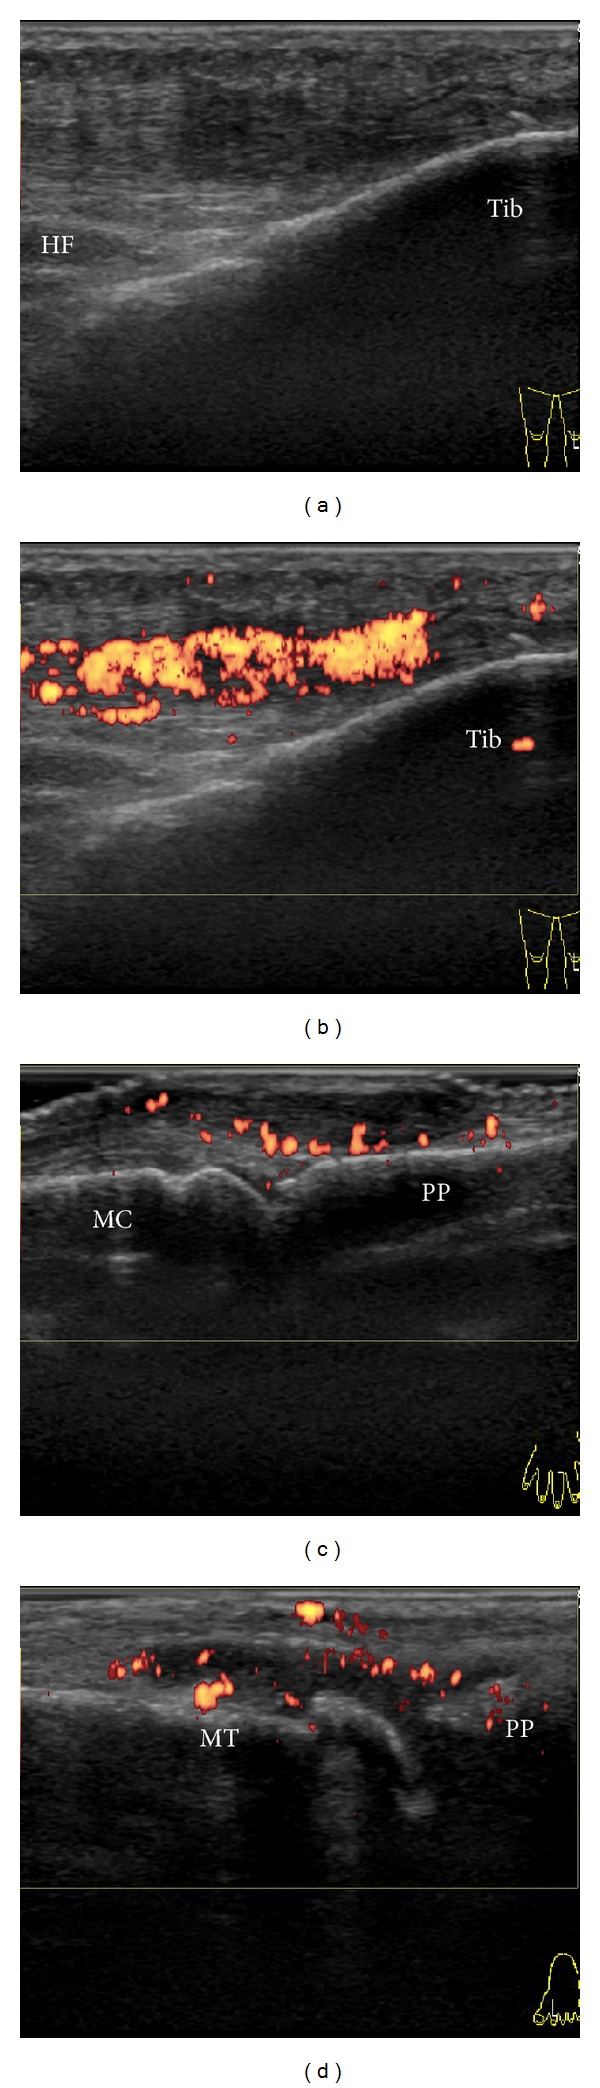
Ultrasound findings of knees, fingers, and toes. (a) Gray-scale and (b) power Doppler ultrasonograms of the longitudinal scan of the distal right patellar tendon (PT). Similar findings were also observed in the left PT (not shown). (c) Power Doppler ultrasonograms of the longitudinal scan of the extensor tendon and metacarpophalangeal joint of the third finger of the left hand. (d) Power Doppler ultrasonograms of the longitudinal scan of the metatarsophalangeal joint of the second toe of the left foot. HF Hoffa's fat pad, Tib Tibia, MC metacarpal bone, PP proximal phalange, MT metatarsal bone.
